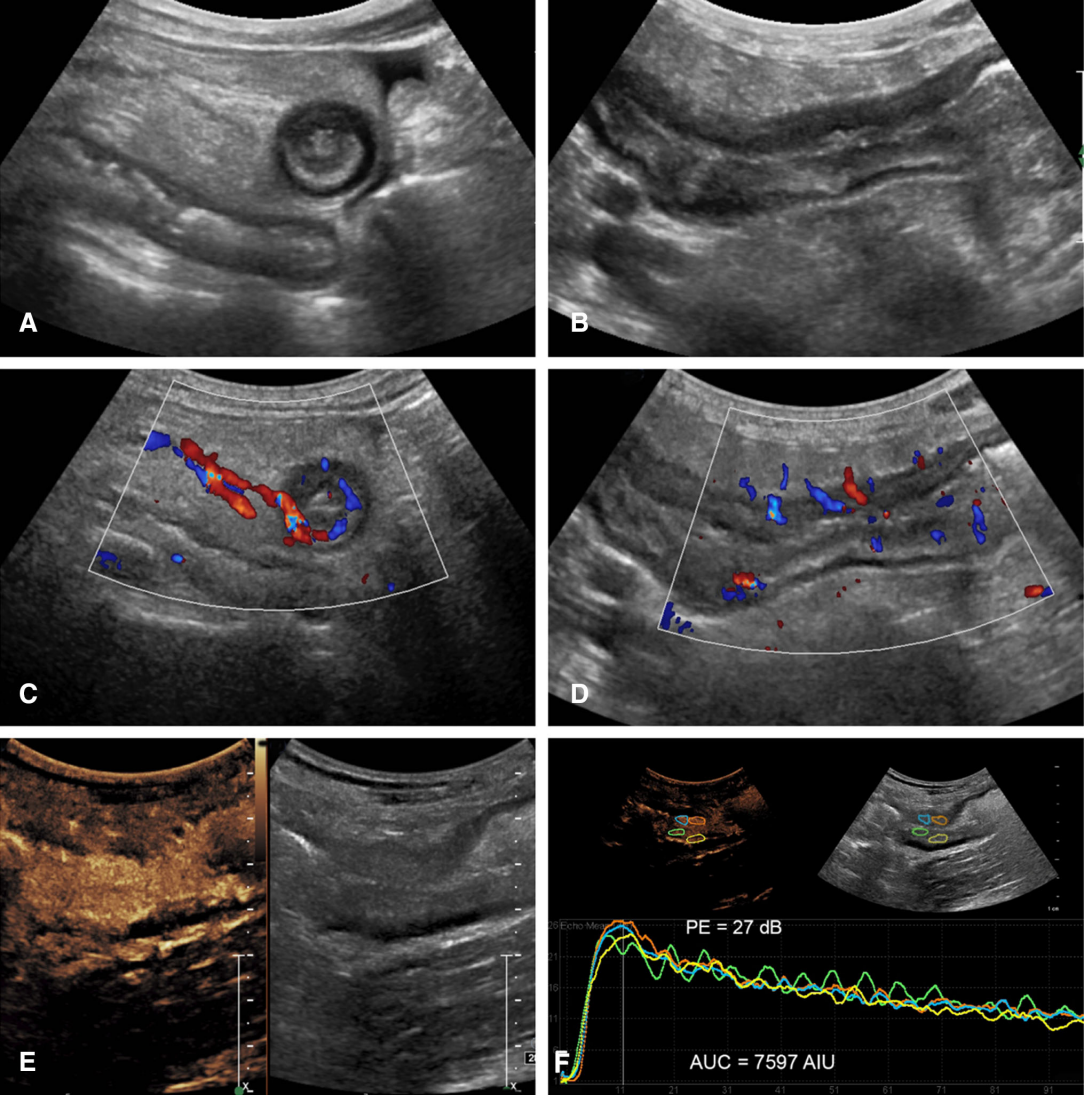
A 41-year-old female with severe active Crohn’s disease of a long segment of the neoterminal ileum. A Axial B and sagittal images of a thickened segment with a large amount of surrounding echogenic inflammatory fat and trace of free fluid. Wall thickness is 1 cm with some loss of the bowel layers. C Axial and D Sagittal color Doppler images show significant wall vasculature and prominent straight mesenteric vessels (a color Comb sign). E Dual-screen CEUS display of the abnormal segment shows transmural hyperenhancement on the left, and a low MI grayscale image for reference on the right. F Generated time–intensity curves from 4 ROIs placed on the anterior and posterior bowel walls (colored circles) show similar curves with high-enhancement values (PE 26 dB) during wash-in followed by very slow progressive decline of contrast enhancement overtime with a large area under the curve. The curves generated from the anterior and posterior wall are essentially equivalent
